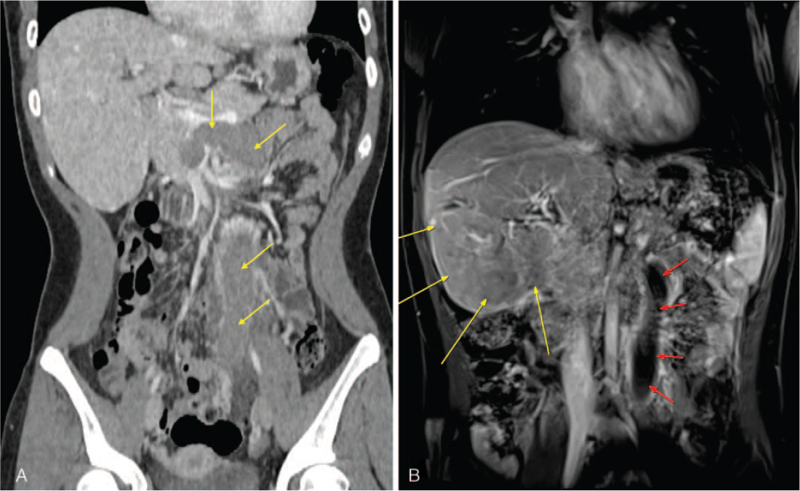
(A) Initial computed tomography with contrast showing inferior mesenteric vein (IMV) thrombosis in the portal phase (4 thin arrows). (B) Initial abdominal magnetic resonance image displaying nodular regenerative hyperplasia lesion (long thick arrows) and IMV thrombus (short, thin arrows).
